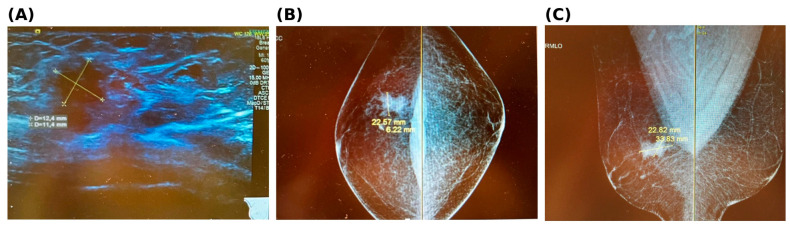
Imaging findings of the right breast lesion. (A) Ultrasonography demonstrating an irregular hypoechoic lesion measuring approximately 12.4 × 11.4 mm with poorly defined margins. (B) Mammography in the craniocaudal (CC) view, showing a high-density lesion measuring approximately 22.6 mm in greatest in-plane dimension. (C) Mammography in the right medio-lateral oblique (RMLO) view, showing the same lesion measuring approximately 33.8 mm in greatest in-plane dimension, corresponding to a maximum mammographic extent of approximately 34 mm.
